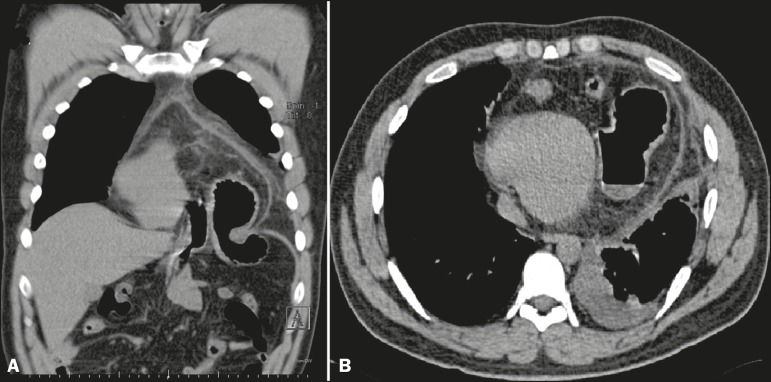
Computed tomography of the chest. Coronal reconstruction (A) and acquisition
